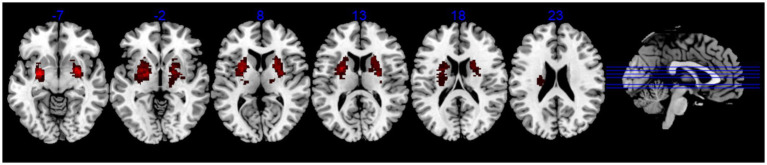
The lesion overlap map of BBGIS patients. Lesion overlap map (warm tones) showing consistent involvement of bilateral basal ganglia and internal capsule across patients, confirmed by MRI/CT. Overlapping regions (thresholded at >50% of participants) highlight the study’s focus on bilateral motor pathway disruptions. MNI coordinates are provided for reference.
